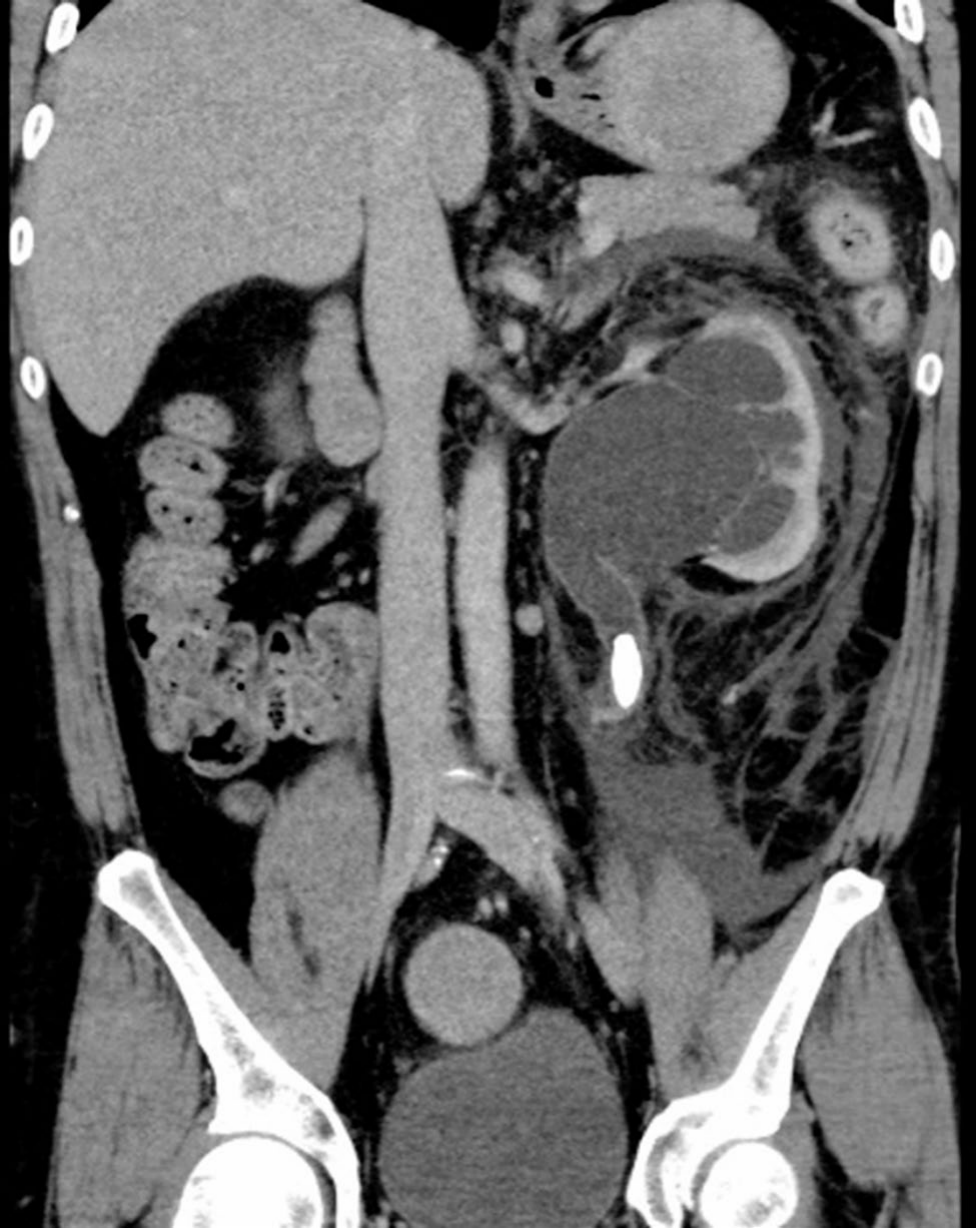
Preoperative computed tomography showing a proximal left ureteral calculus with moderate hydronephrosis
